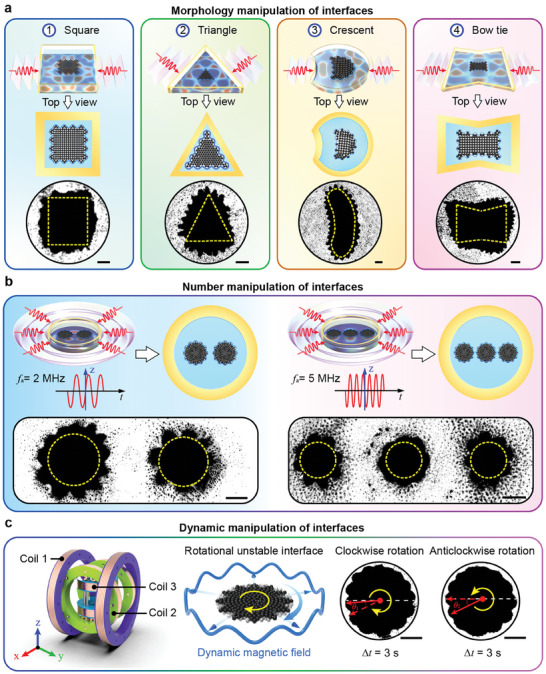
Static and dynamic manipulation of the unstable interfaces. a) Morphology manipulation of the unstable interface (squares, triangles, crescents, and bow ties) by changing the shape of the polyimide tape. Images showing the unstable interface and morphology of swarms under different acoustic fields and the oscillating magnetic field. Scale bar: 200 µm. b) Number manipulation of the unstable interface within a single experimental cell. The height of the polyimide tape used in the experiment was *h* = 375 and 150 µm, respectively. The ultrasonic frequency was *f*
